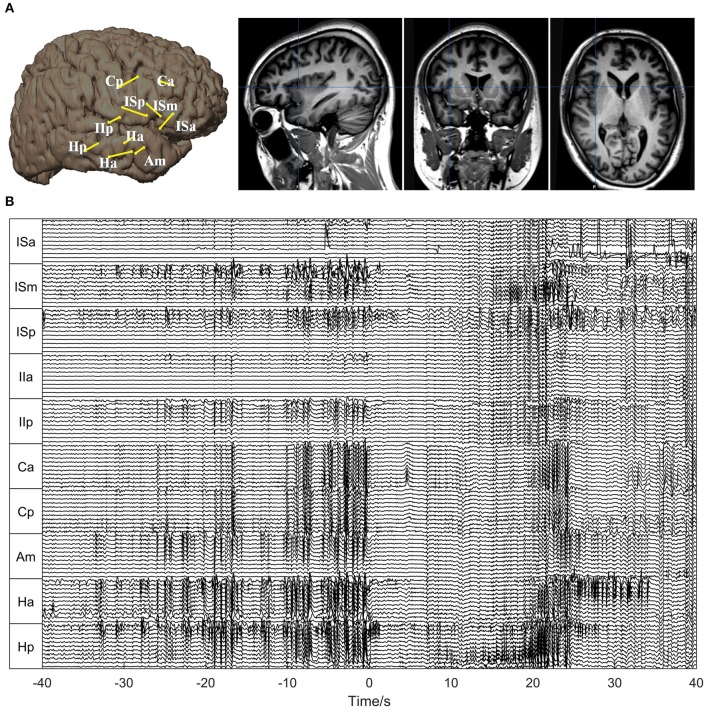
Electrode placement and SEEG signals for Patient 1. (A) Electrode placement. The left panel shows the electrode trajectories on the 3D brain schemes. The right panel shows the trajectory of the electrode Ha superimposed on the sagittal, coronal, and axial MRI views, respectively. (B) The SEEG signals during one seizure. The seizure onset time is set as 0. As can be seen, the seizure started with typical low-amplitude fast activities, preceded by interictal (pre-ictal) spikes.
